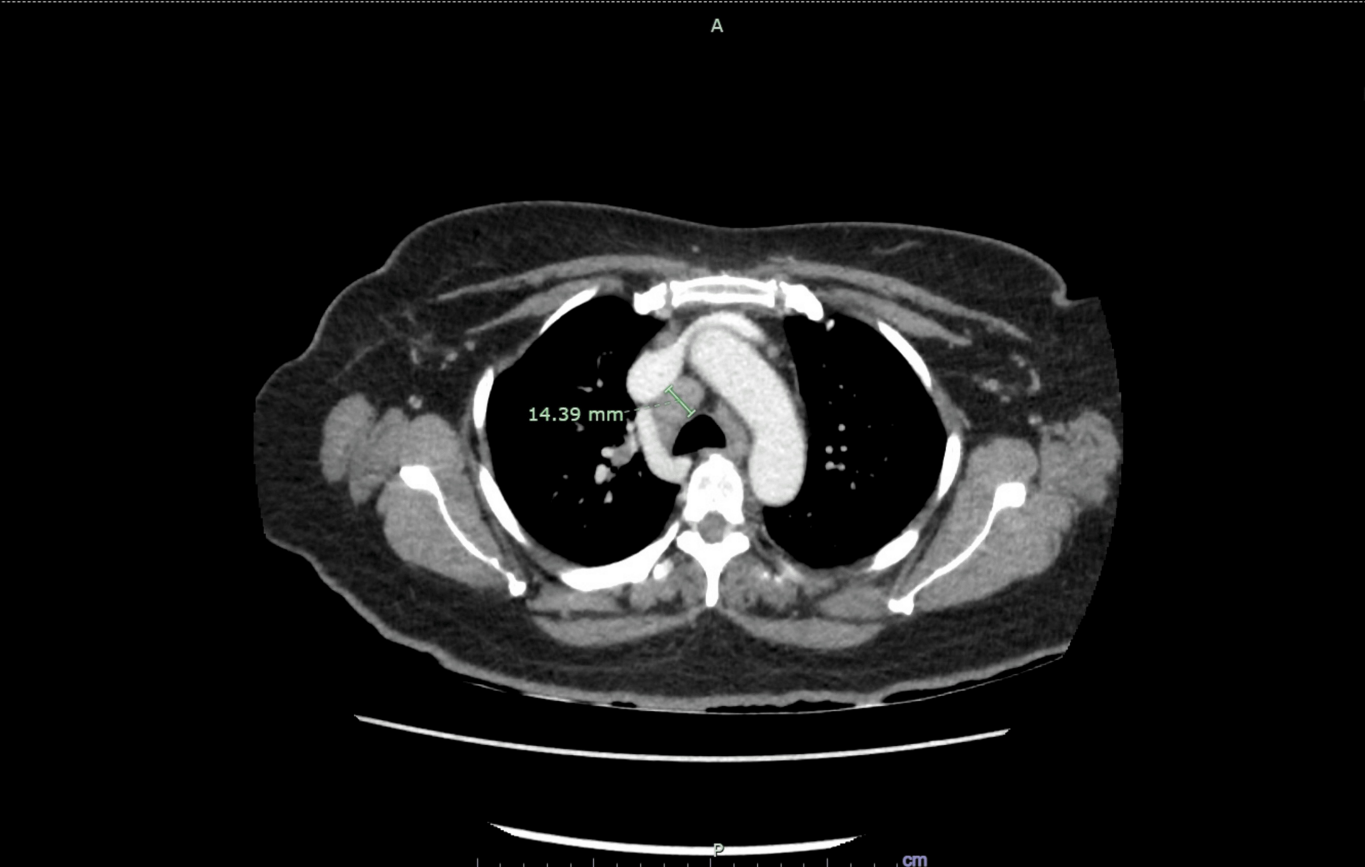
Axial CT of the chest with intravenous contrast showing mediastinal lymphadenopathy (green arrow). CT, computed tomography
